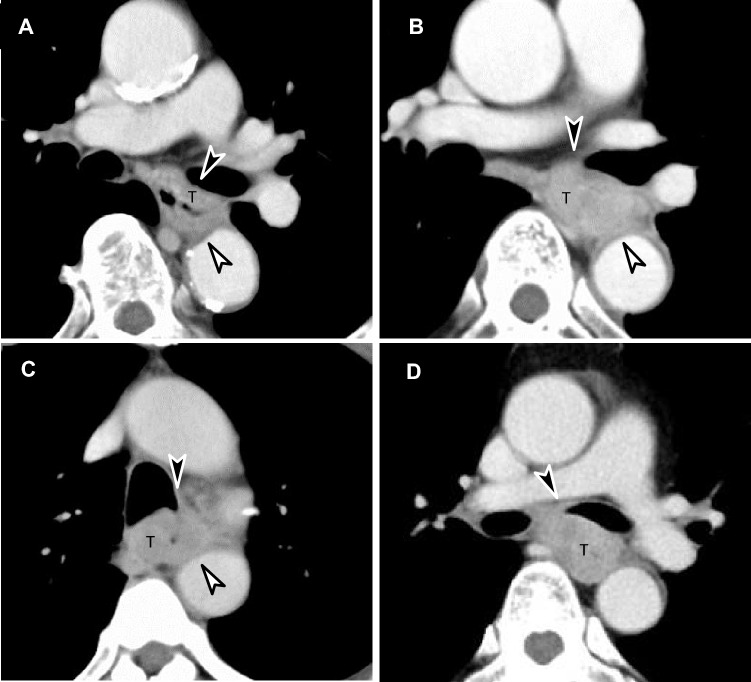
Images of borderline resectable locally advanced esophageal cancer (BR-LAEC) on initial conventional CT (i-CT). Axial contrast-enhanced portal venous phase CT images at the level of the thoracic esophagus (a–d). The black arrowhead represents the suspected site of the main bronchial invasion, and the white arrowhead represents the suspected site of aortic invasion. a BR-LAEC with 100 mm of craniocaudal length in a 77-year-old man, with the tumor slightly surrounding the bronchus without compression (Br-BR1) and surrounding the aorta, but not widely (Ao-BR1). b BR-LAEC with 45 mm of craniocaudal length in a 60-year-old man, with the tumor slightly surrounding the bronchus with compression (BR-BR2) and surrounding the aorta widely (Ao-BR2). c BR-LAEC with 60 mm of craniocaudal length in a 63-year-old man, with a tumor highly surrounding the bronchus (Br-BR3) and surrounding the aorta widely (Ao-BR2). d BR-LAEC with 40 mm of craniocaudal length in a 56-year-old man, with the tumor highly surrounding the bronchus (Br-BR3). The result of 4DCT is that the first three cases are all resectable (a, b, c), and the last case is unresectable (d)
